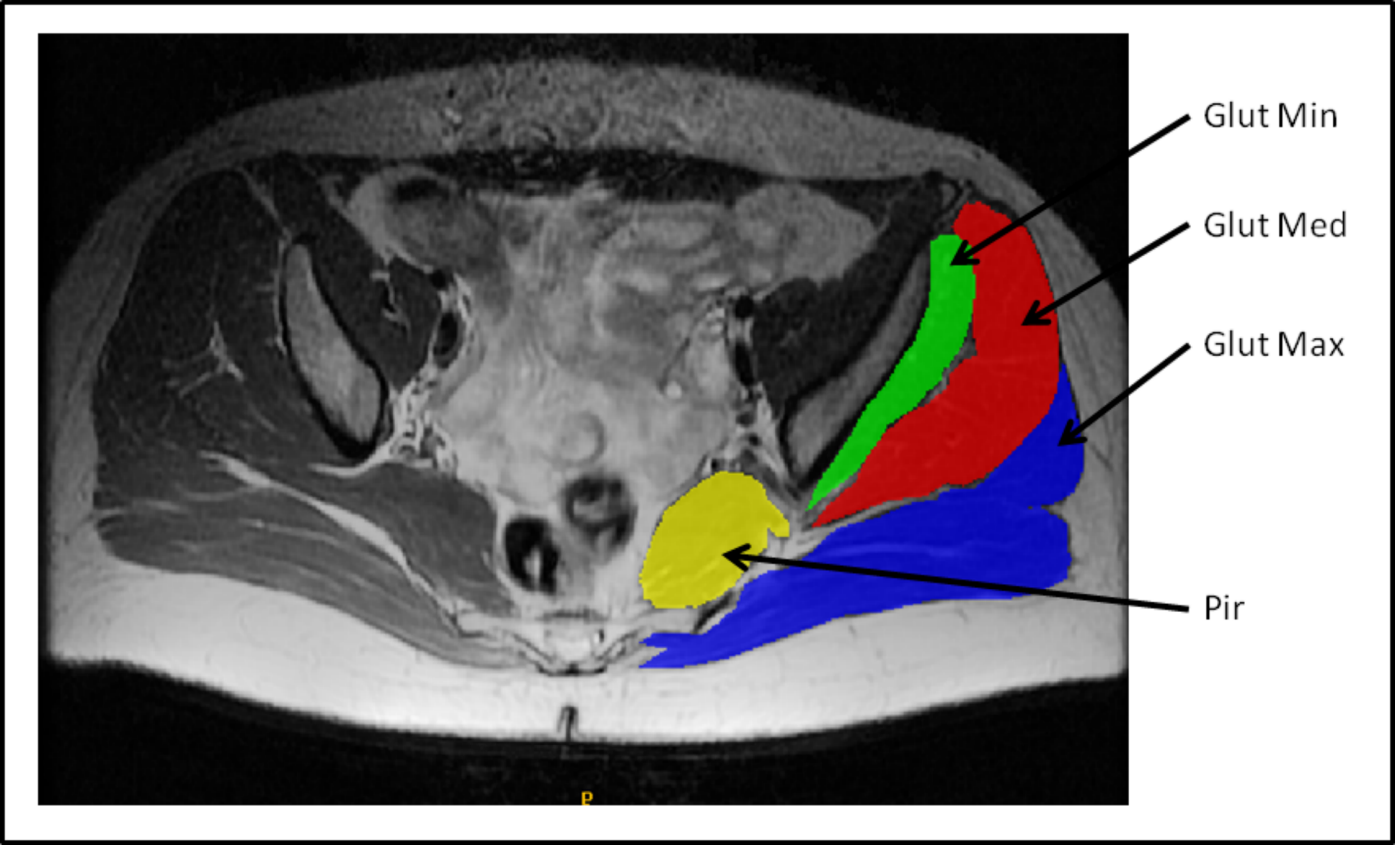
Sample of a single MRI slice with final segmentation of the four chosen muscles. Legend: Glut min–gluteus minimus muscle, Glut med–gluteus medius muscle, Glut max–gluteus maximus muscle, Pir–piriformis muscle. All anatomical objects defined by closed contours were filled in by selected colors.
